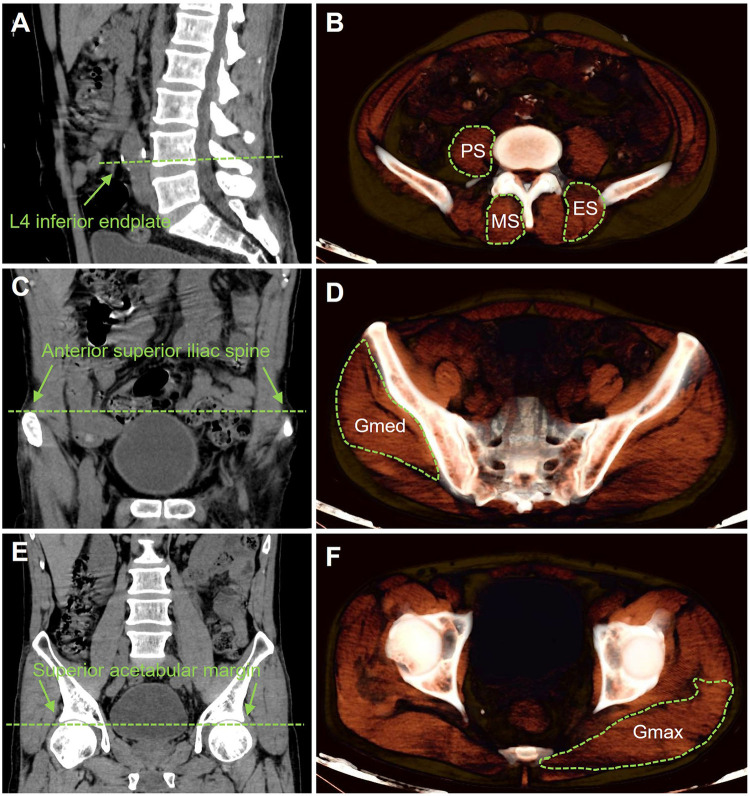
Measurement of cross-sectional area for core muscle morphology. (A) Sagittal CT image at the level of the inferior endplate of L4. (B) Corresponding axial cross-sectional area for psoas major, multifidus, and erector spinae muscles. (C) Coronal CT image at anterior superior iliac spine level. (D) Corresponding axial cross-sectional area for Gluteus medius muscles. (E) Coronal CT image at upper-acetabular margin level. (F) Corresponding axial cross-sectional area for gluteus maximus muscles.
